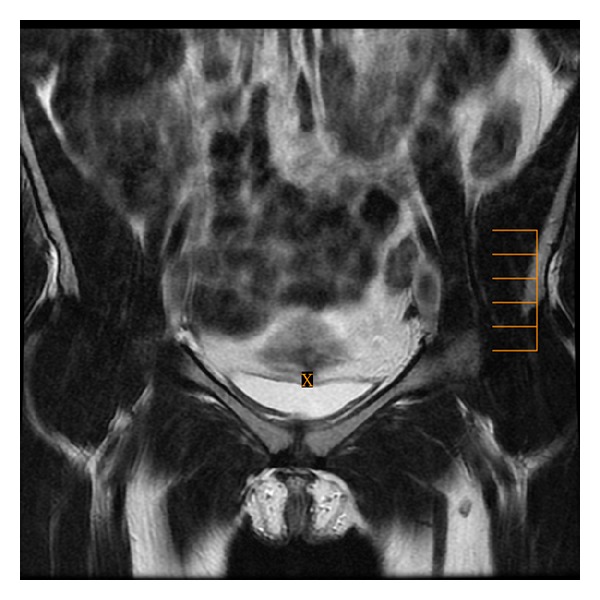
Coronal T2-weighted fast SE image (repetition time msec/echo time msec = 3020/101) shows the vesicouterine pouch, the potential site of anterior endometriosis.
